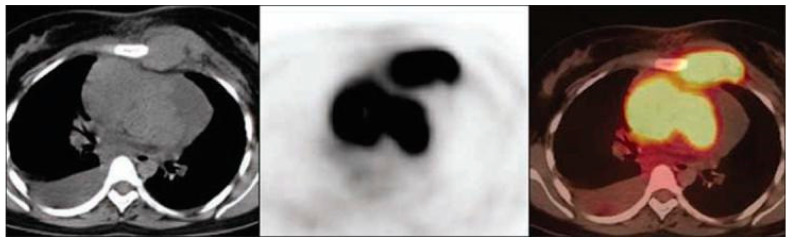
Axial images from non-contrast CT, PET, and PET-CT demonstrate elevated FDG uptake in the mediastinal and the left internal mammary lymph nodes in a patient diagnosed with lymphoma. Source: [17].
